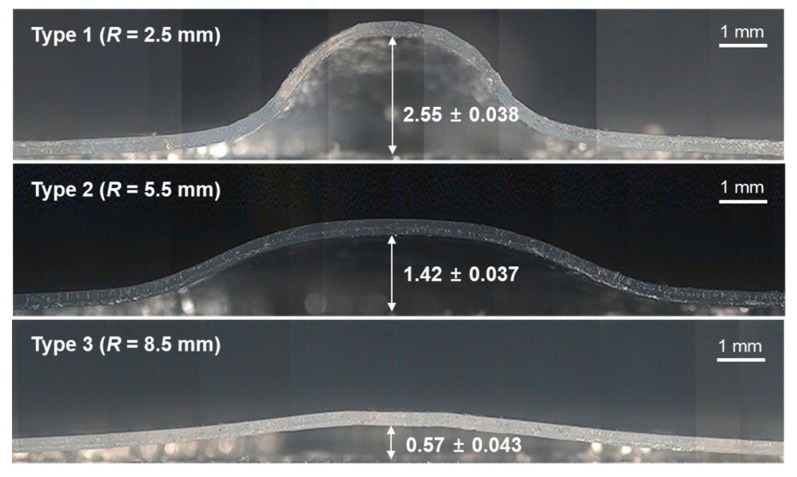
Sectional photographs of the formed film after ultrasonic stretching using various horn types with different tip radii (unit: mm).
